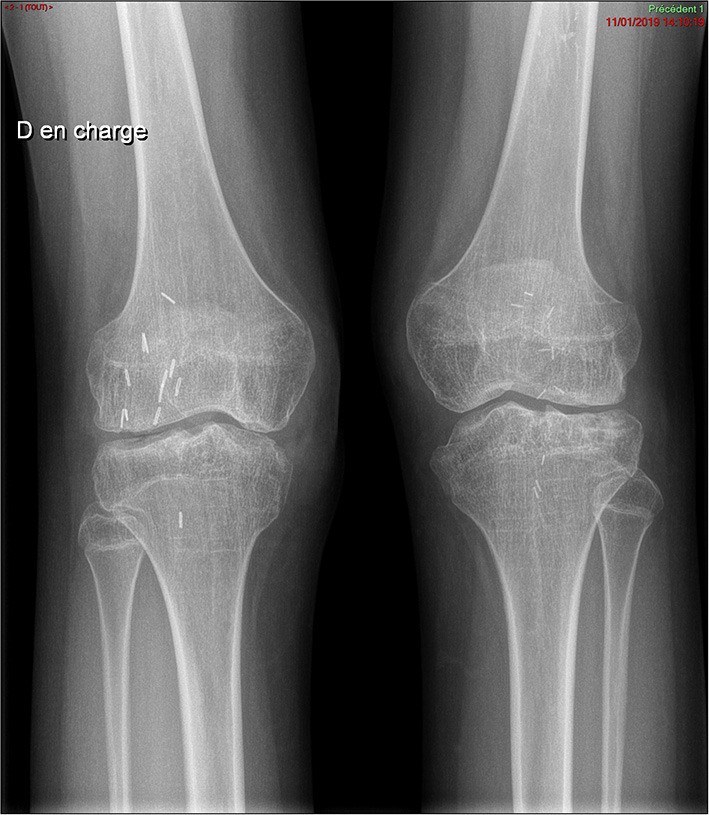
Plain x-ray of the knees. Pancompartmental joint space loss reflecting secondary osteoarthritis changes, with presence of diffuse joint narrowing and subchondral cysts.
